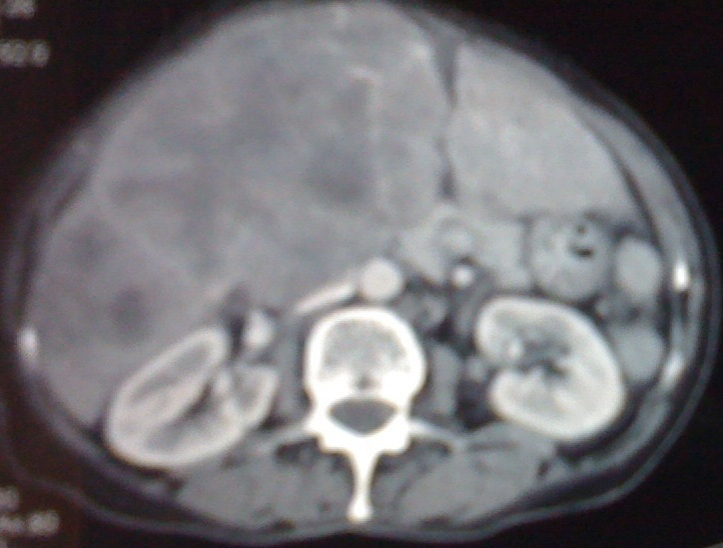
Liver computed tomography showing multiple diffuse metastatic lesions.
